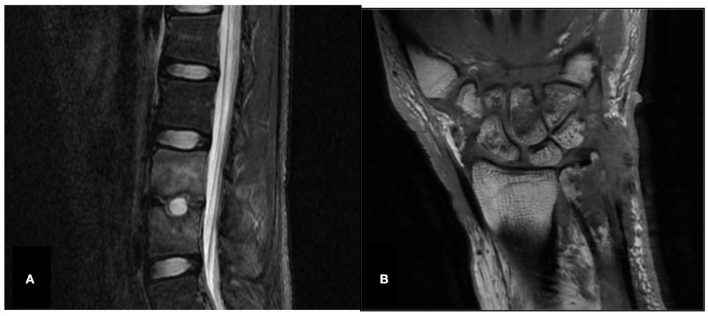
Spine and joint MRI (Case 10 and Case 18X). (A) Case 10, spinal magnetic resonance imaging (MRI), abnormal signals in the L3 and L4 vertebrae, abscess, narrowing intervertebral space and disappearance of the normal disc signal. (B) Case 18X, left wrist joint MRI, left distal ulna, multiple carpal and metacarpal bone destruction, left wrist joint soft tissue swelling.
